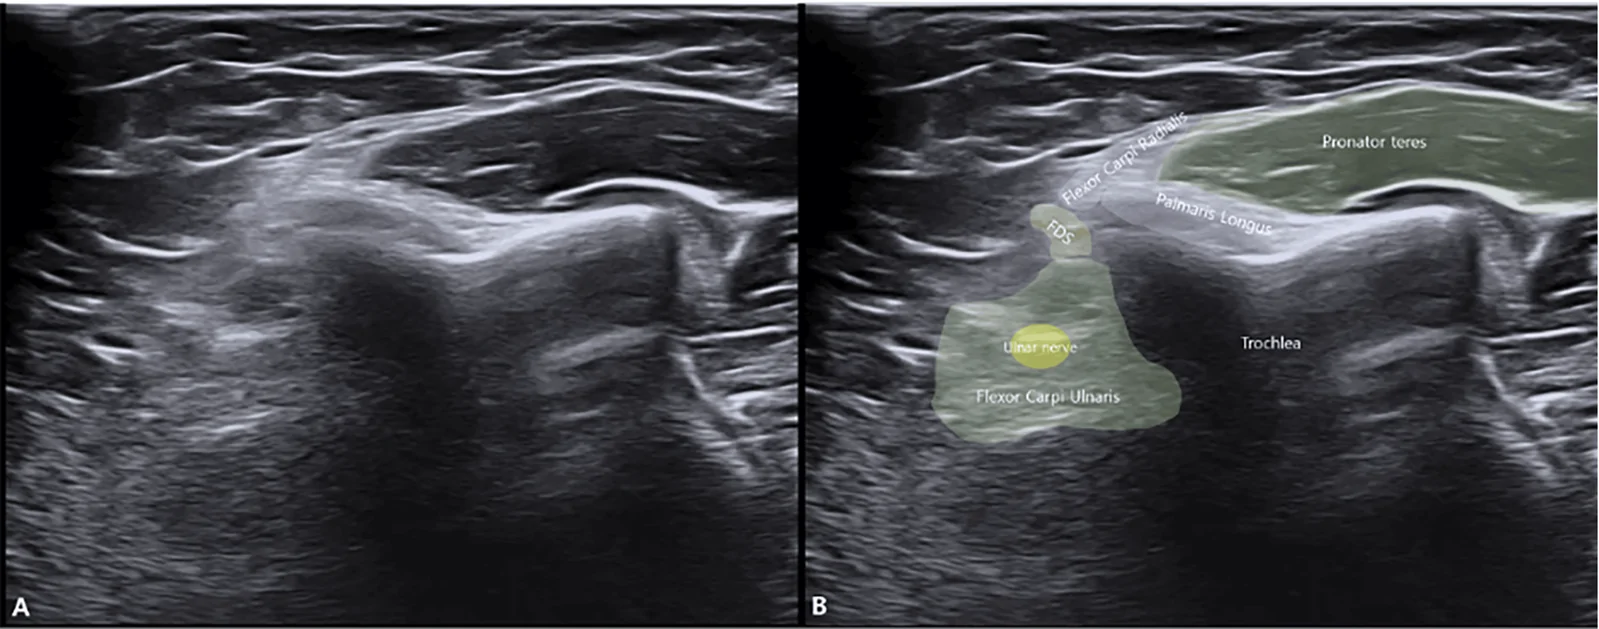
Short-axis dynamic ultrasound showing a symptom-correlated snap or shift in the proximal FDS region Short-axis ultrasound image obtained near the medial elbow at the symptomatic region, approximately 1 cm distal to the proximal FDS origin. During active finger motion, a reproducible snap or shift involving tissue within or immediately adjacent to the proximal FDS was observed at this site. This finding was temporally associated with the patient's characteristic triggering sensation and is presented as a proposed pathoanatomical explanation rather than a definitive diagnosis. FDS, flexor digitorum superficialis Image courtesy: Yonghyun Yoon and King Hei Stanley Lam
